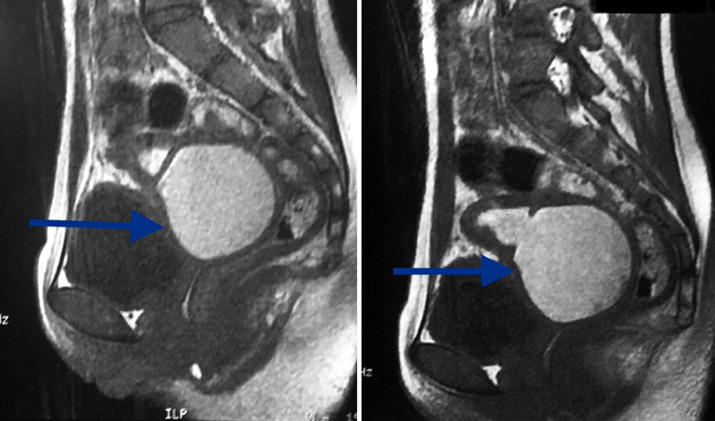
MRI appearance in T2 sequence of vaginal atresia with hematometrocolpos
